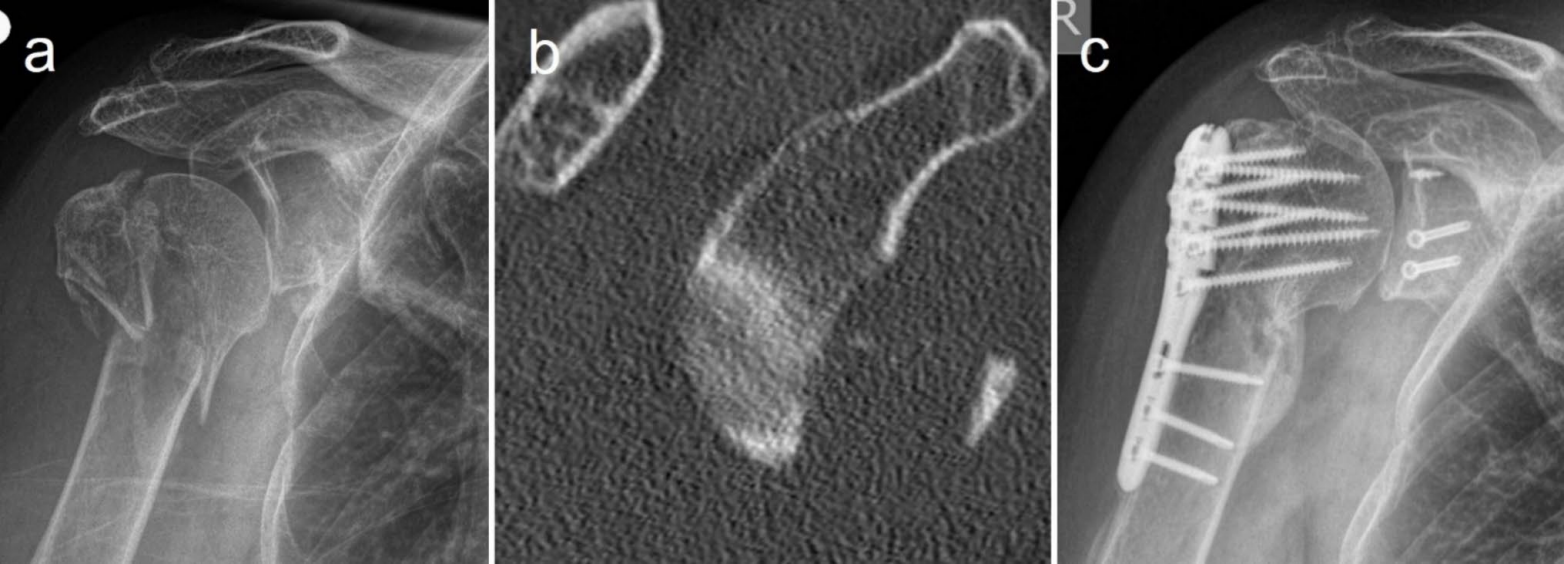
Glenohumeral combination fracture in a 72-year-old female with a large and displaced glenoid rim fracture (a.p.-radiograph, b CT scan sagittal plane) indicated for surgery. Image c (a.p.-radiograph) shows the postoperative result within further course with prolonged bony consolidation and an excellent clinical result (CS 78, WOSI 82, OXSC 41, RS 80)
